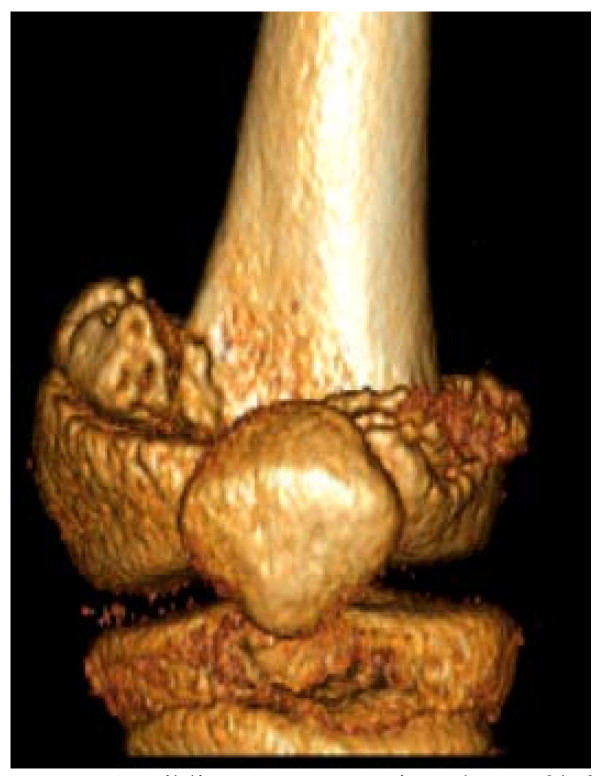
Sibling A - Reconstituted CT of left distal femur collapse/impaction, aged 10.
